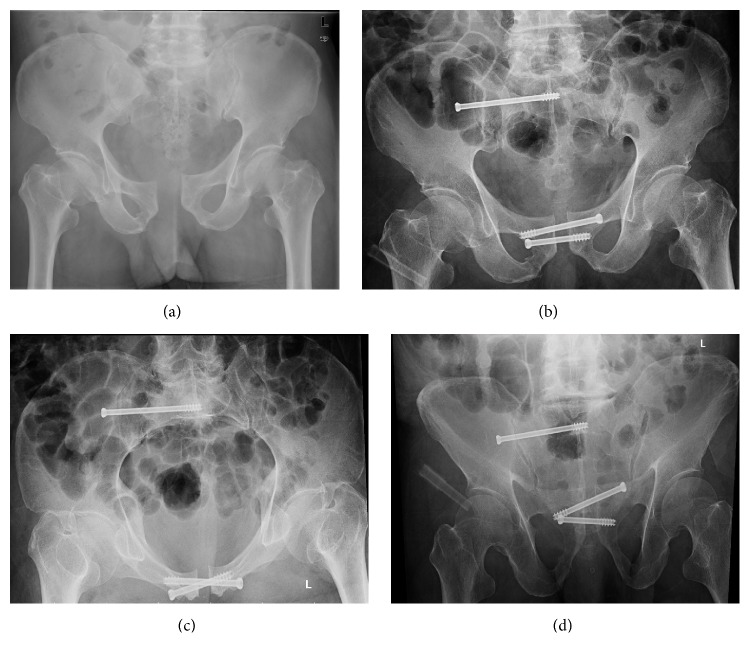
Case number 1. (a) Preoperative AP view radiograph; (b), (c), and (d) postoperative AP, inlet and outlet view radiographs.
